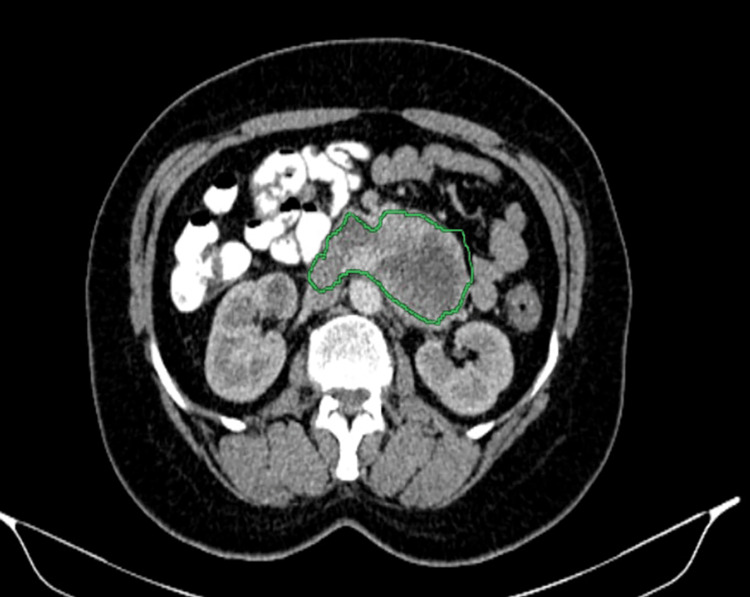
The axial CT scan of the abdomen shows a well-defined mass near the pancreas (outlined in green). The mass was diagnosed as a GIST involving the duodenum. GIST: Gastrointestinal stromal tumor
